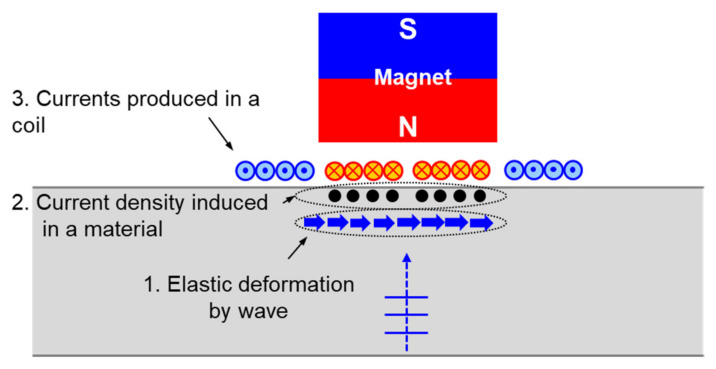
Ultrasonic wave receiving mechanism of the EMAT used in the experiments.
